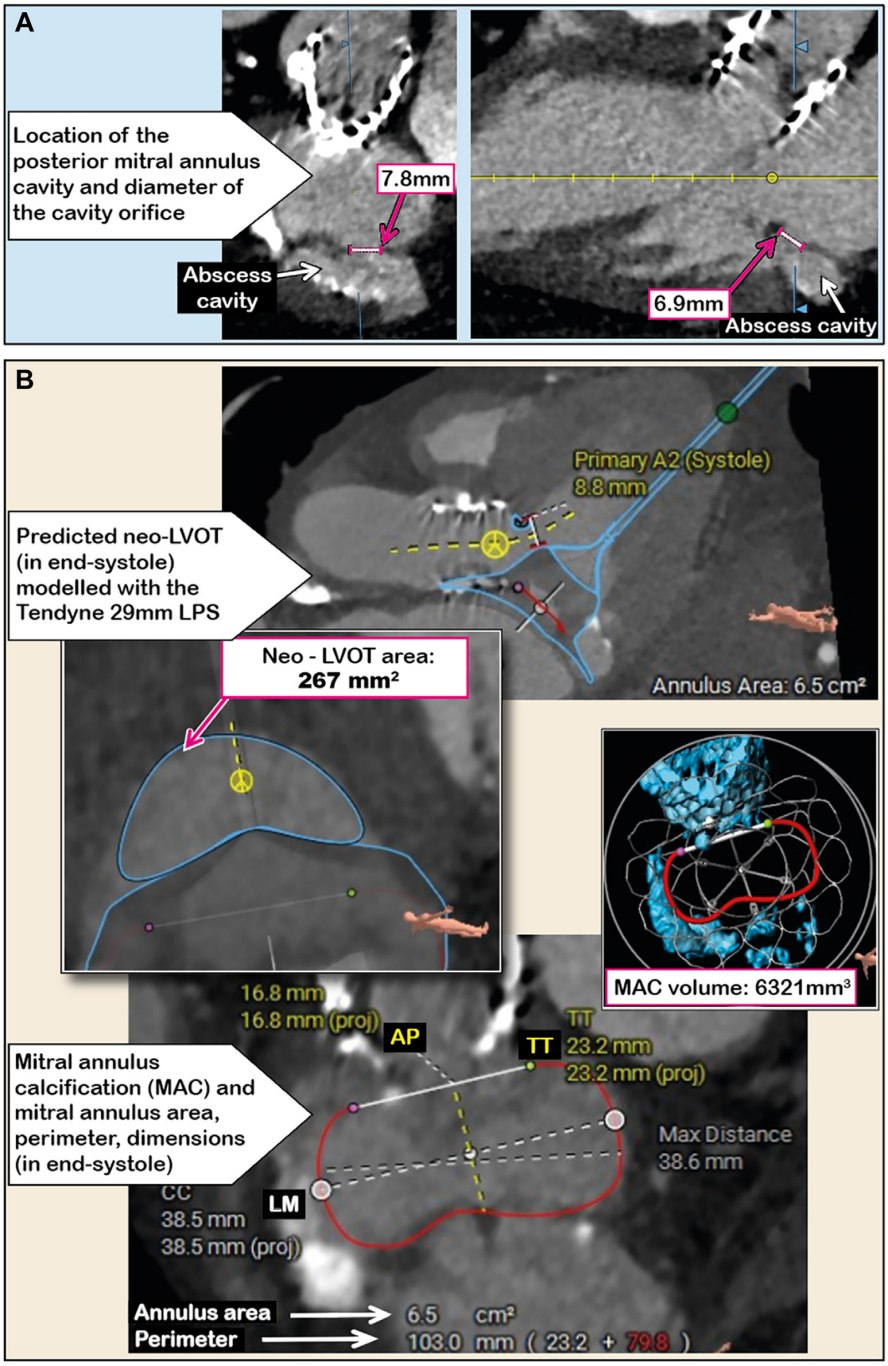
Pre-Procedure Computed Tomography Imaging (A) Positron emission computed tomography performed 1 month preprocedure, showing the location of the posterior mitral annular abscess cavity and diameter of the cavity orifice. (B) Preprocedural multislice computed tomographic evaluation in the end-systolic cardiac phase. Neo–left ventricular outflow tract (LVOT) area was 267 mm^2^; mitral annular anteroposterior (AP) diameter excluding the cavity was 16.8 mm; lateral-medial (LM) diameter was 38.5 mm; intertrigonal (TT) distance was 23.3 mm, area 6.5 cm^2^, and perimeter 103 mm; and moderate mitral annular calcification (MAC) was present (volume 6,321 mm^3^). LPS = low profile system.
